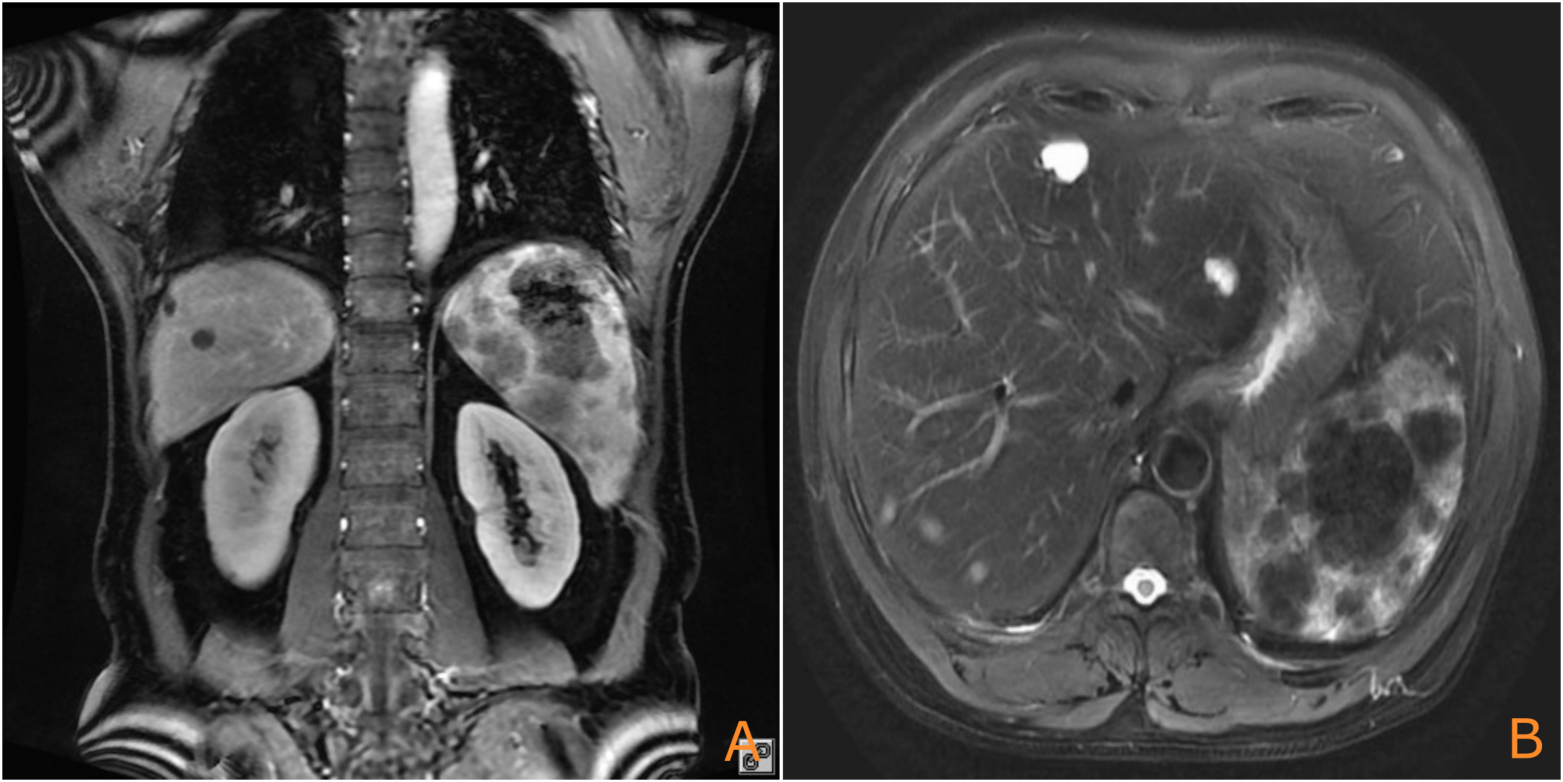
Prior MRI (August 2022) showing the splenic mass (arrow), which was not intervened upon [(A): coronal plane; (B): axial plane].
